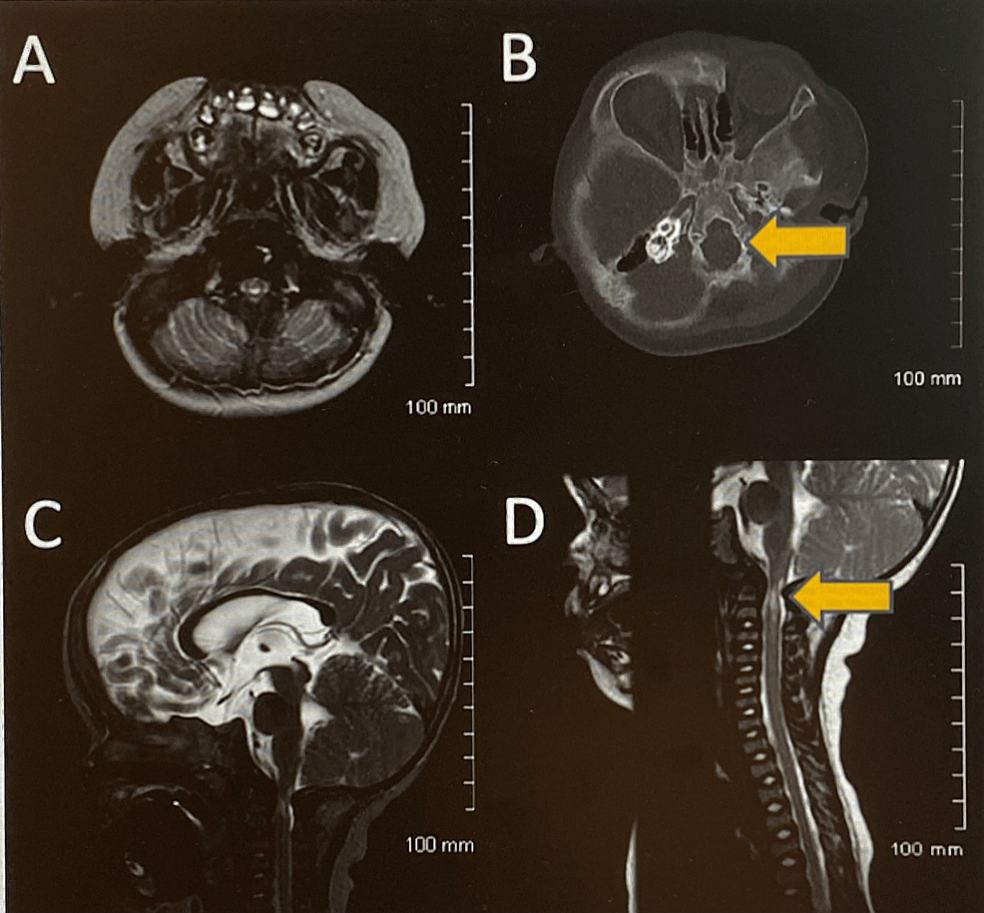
MRI and CT head and neck findings Images A (axial T2 MRI), C (sagittal T2 MRI), D (sagittal T2 MRI) - stigmata of achondroplasia with moderate-to-severe foramen magnum narrowing with kinking of the cervicomedullary junction with patchy T2 hyperintense signal abnormality in the upper cervical spinal cord and cervicomedullary junction, arrow in the sagittal image D. Image B (non-contrast CT) - small skull base with narrowing of the foramen magnum, arrow in the image. MRI, magnetic resonance imaging; CT, computed tomography
